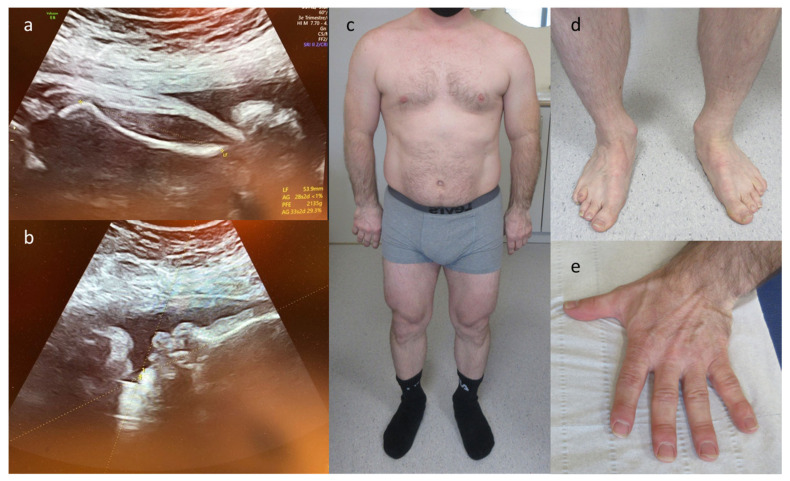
Phenotype of fetus and father with Stickler syndrome due to a *COL2A1* variant. (a) 3rd trimester ultrasound showing femoral shortening and bowing; (b) 3rd trimester ultrasound showing pathological lower facial angle at 44° corresponding to microretrognathia; (c) Affected father: short and stocky appearance; (d) Feet of affected father; (d) Feet of affected father: right 4th toe and left 4th and 5th toes are proximally implanted and the lower limbs show bowing at the ankles; (e) Left hand of affected father: short hand and brachydactyly.
